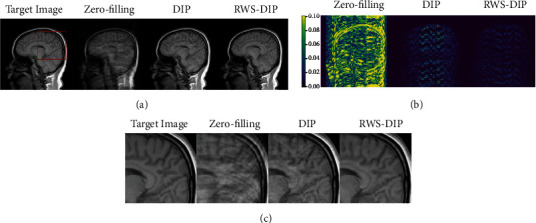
Comparison of reconstructions of the target MR image in Brain A using Cartesian undersampled mask with 20% sampling rate: (a) the target image and reconstruction results, (b) the corresponding error images, and (c) the corresponding zoom-in images.
